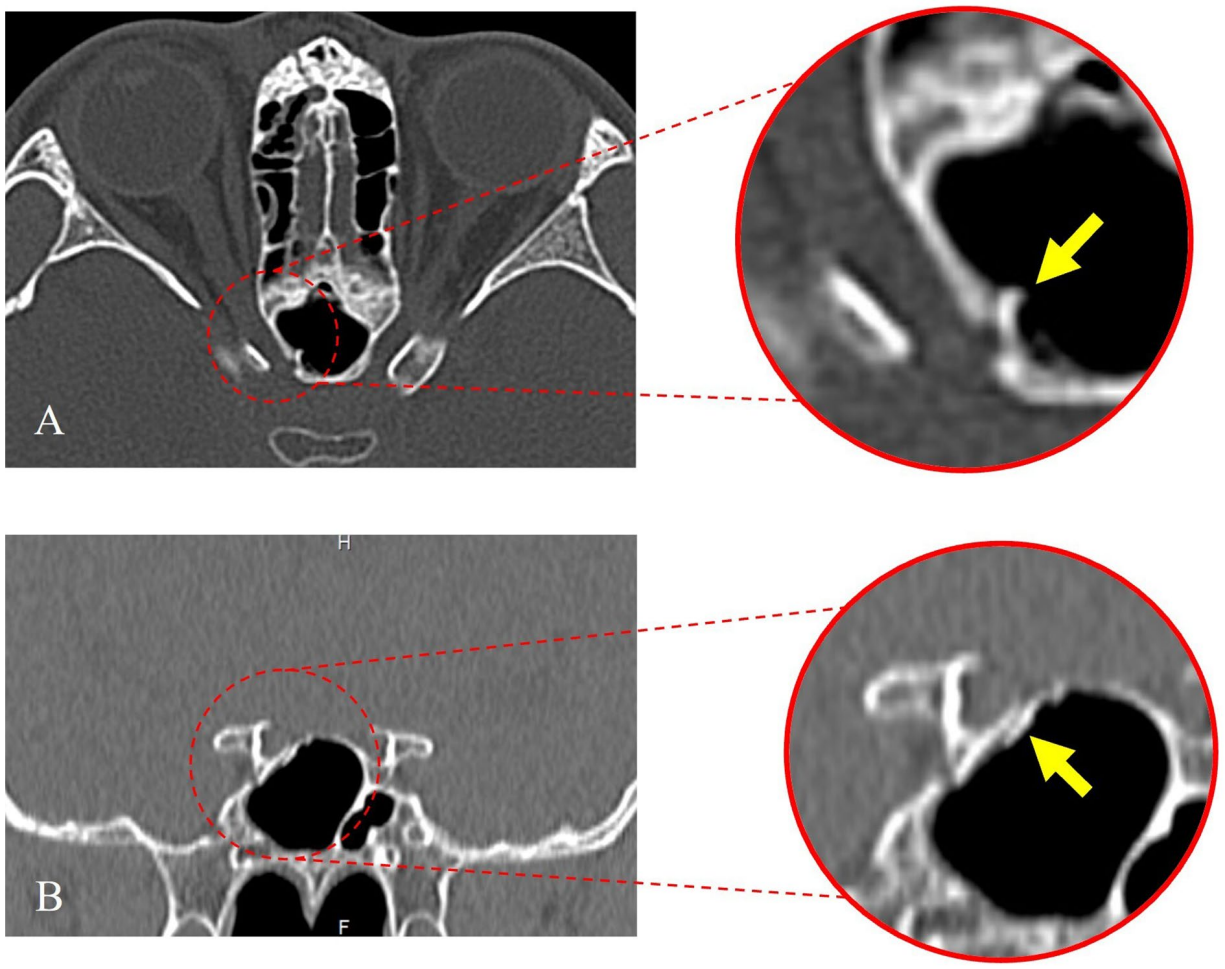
Preoperative orbital computed tomography (CT) thin-slice scan. (A) The axial position of the optic nerve tube shows the fracture line. (B) The coronal position of the optic nerve tube shows the fracture line.
